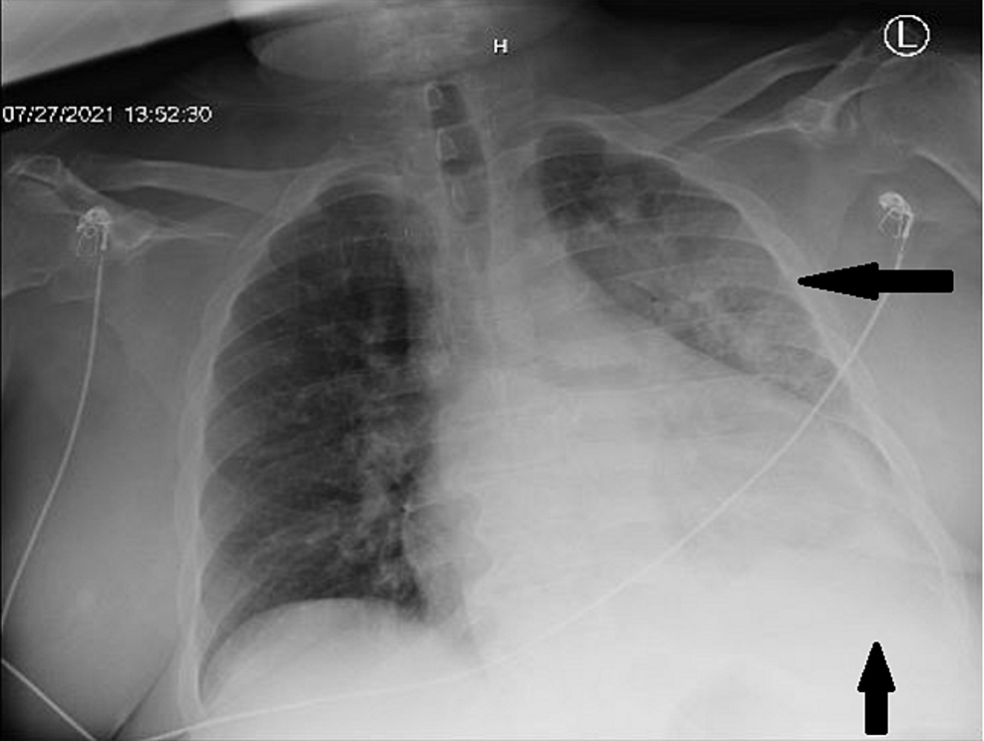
Initial presentation on chest x-ray showing asymmetric left lung area of consolidation with small left effusion.
